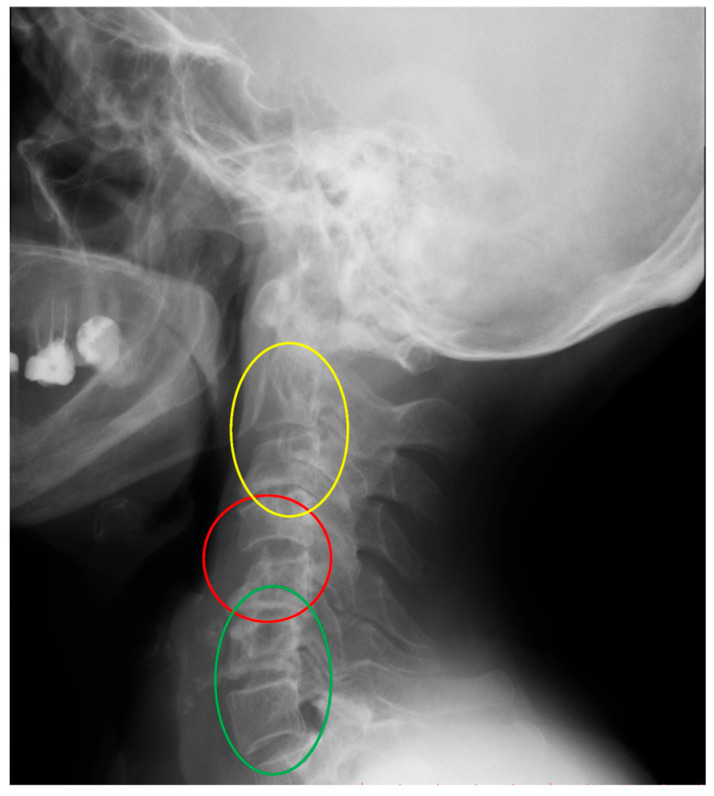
An X-ray of the cervical spine (lateral view) of a 75-year-old female patient with RA and SAS instability. C4–C5 instability (red). Cervical deformity with cervical lordosis below C4–C5 segment (green) and flatneck deformity above C4–C5 segment (yellow).
